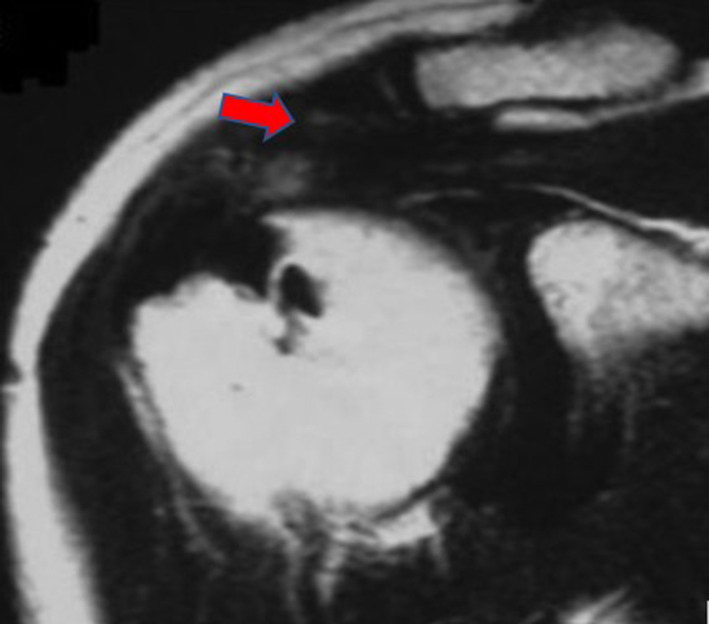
MRI of supraspinatus tear Sugaya type II
